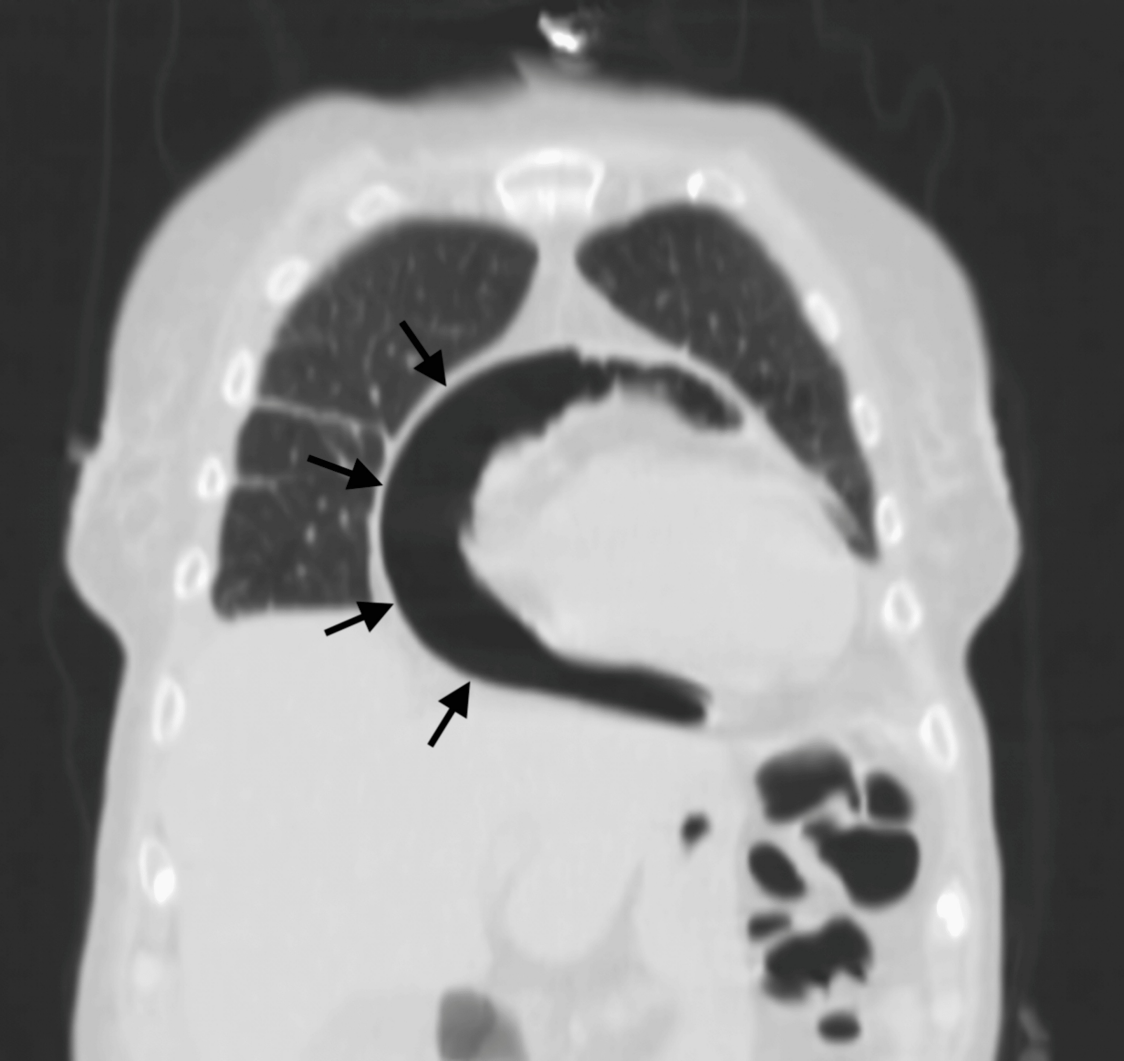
Coronal reconstruction of a contrast-enhanced CT scan in a pulmonary (lung) window. The image demonstrates an extensive purulent pericardial collection enveloping the heart, with a clear air–fluid interface.
